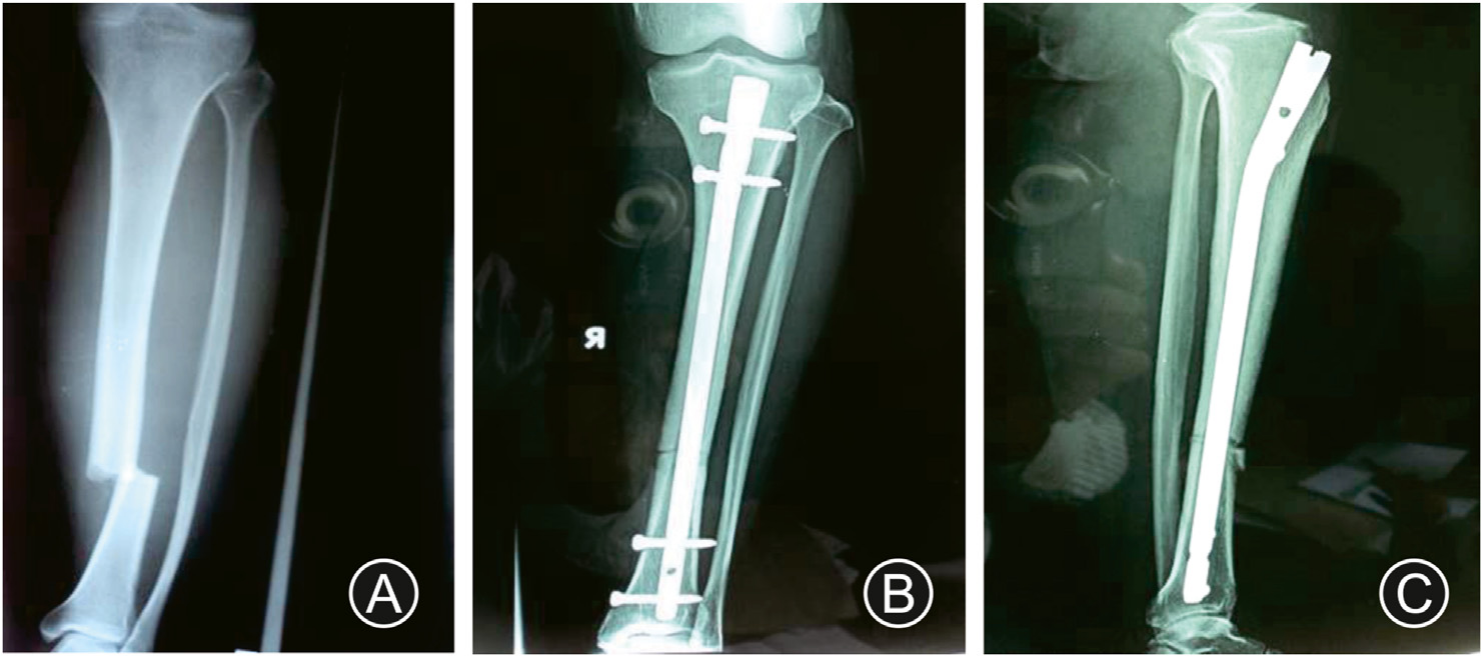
(A) Preoperative radiograph of ankle and leg showing distal third tibia fracture; (B) Postoperative Xray showing IMIL nail 3 months after surgery anteroposterior view; (C) Lateral View.
